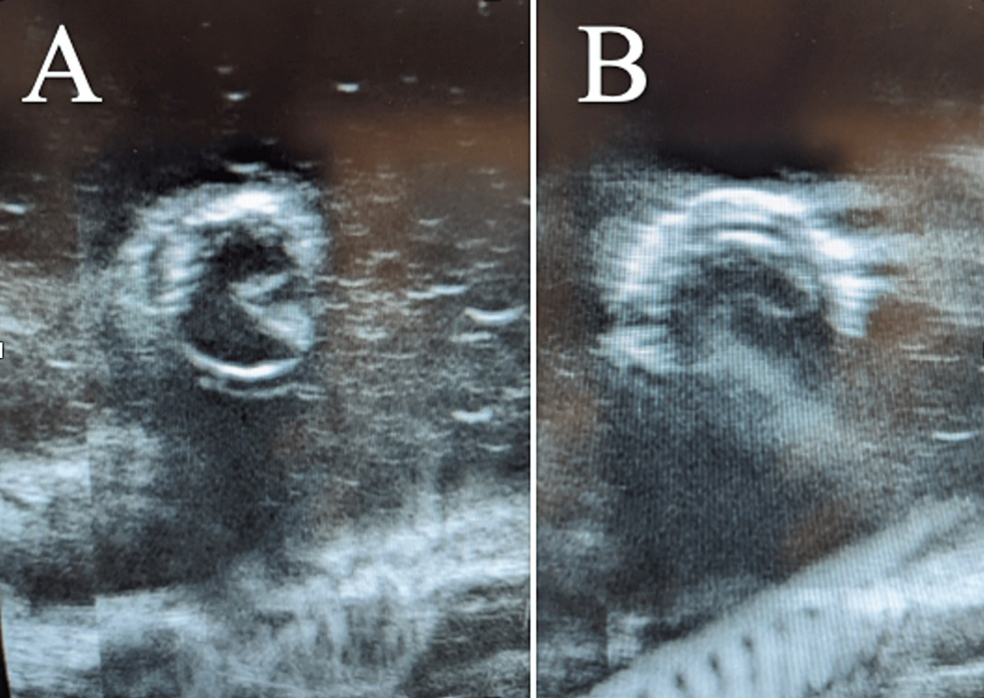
A) Transverse view of the intussusception model visualized by ultrasound. B) Second view of the model at a different point
